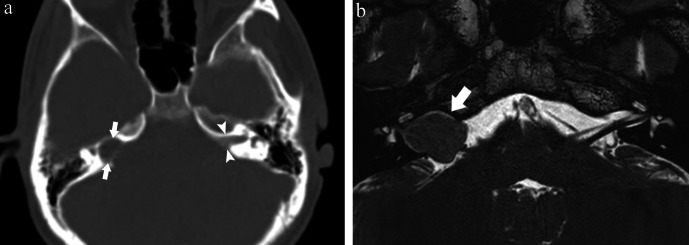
(a) Computed tomography (CT) component of a positron emission tomography
